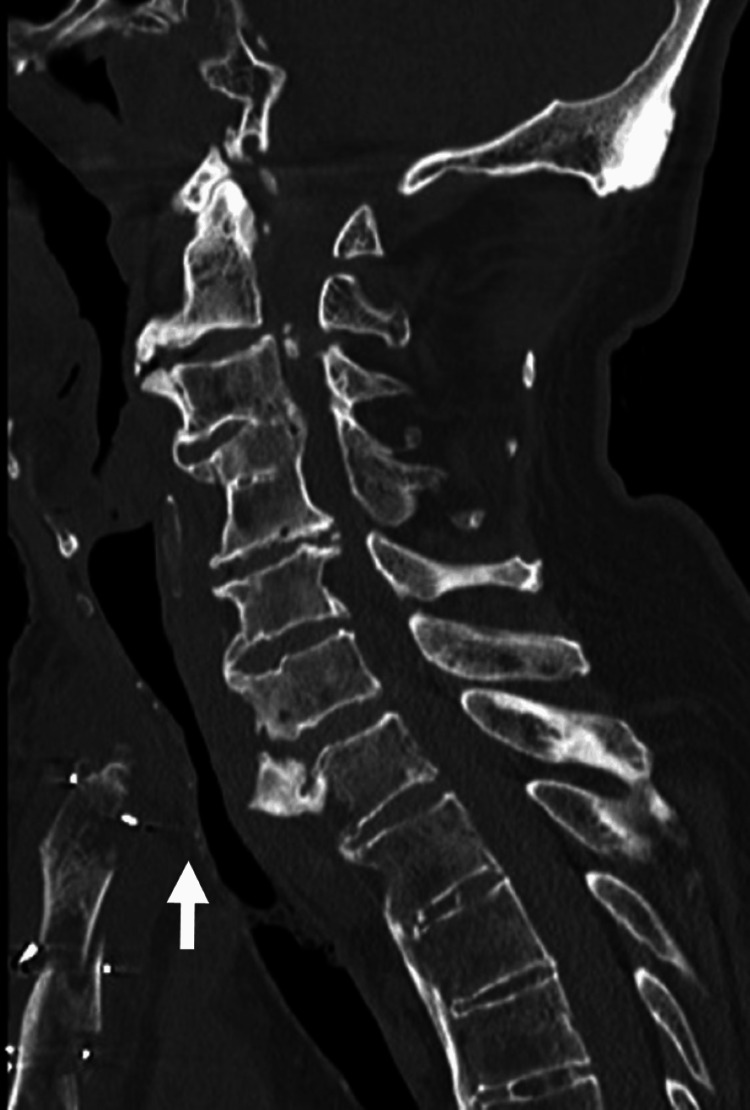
Postoperative CT of the upper thoracic level The arrow indicates that most of the bony overgrowth has been resected, with relief of tracheal stenosis.
